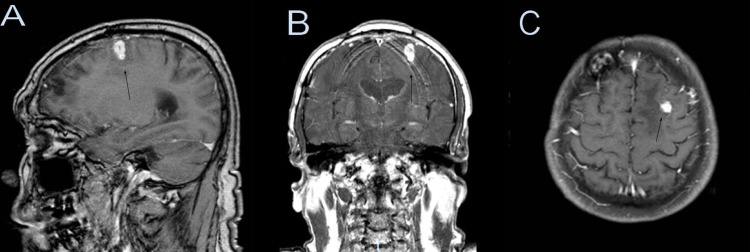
Follow-up brain MRI with and without contrast seven months later, showing that the enhancing mass has reduced (black arrow), measuring 0.7 x 0.7 x 0.7 cm. A central non-enhancing area of 2 mm is noted corresponding to the bone fragment. Sagittal (A), coronal (B), axial (C).
